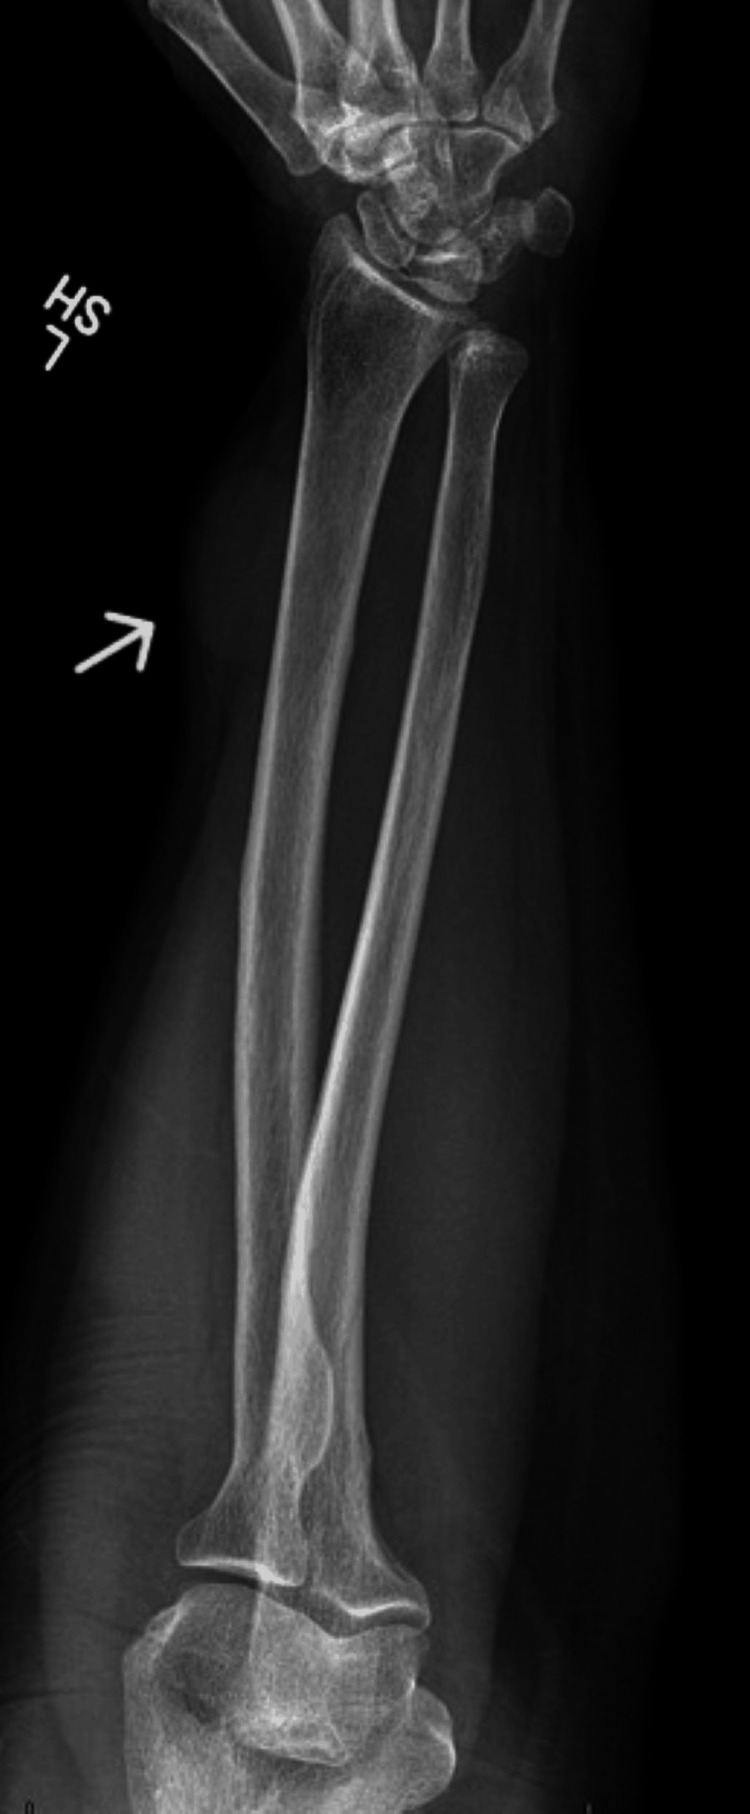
Radiologic imaging. X-ray of left forearm; arrow at mass.
